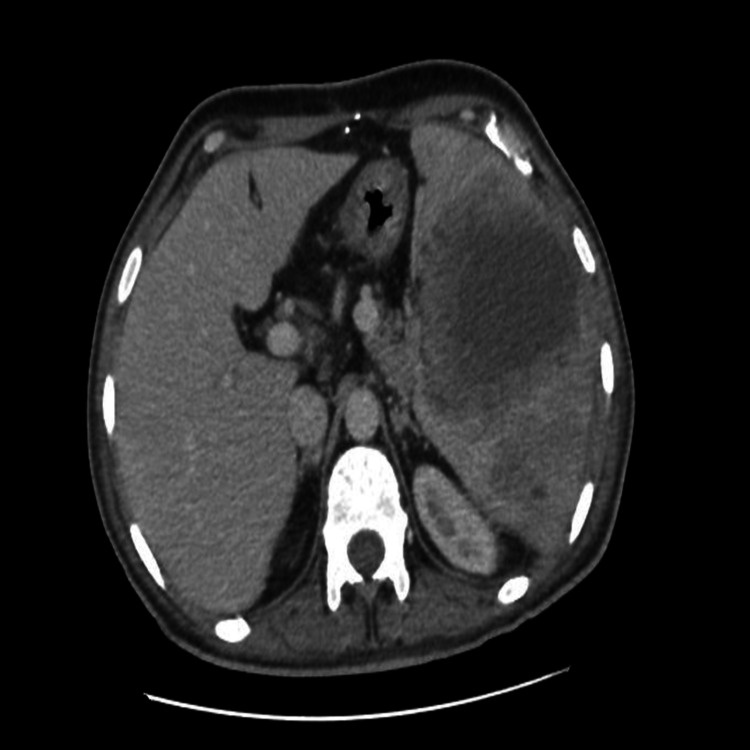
Control CT axial section after antibiotic therapy showing a stable aspect of the splenic abscess CT: computed tomography
